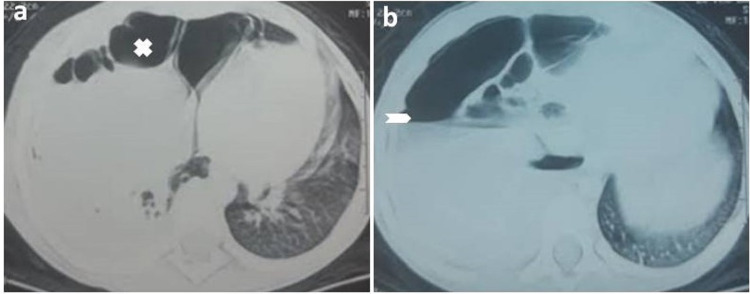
Thoracic CT scan Axial CT scan showing overdistension of the right chest with cystic air-containing lesions (a) with significant hydropneumothorax (b) diagnosed preoperatively as CCAM. CCAM: congenital cystic adenomatoid malformation.
